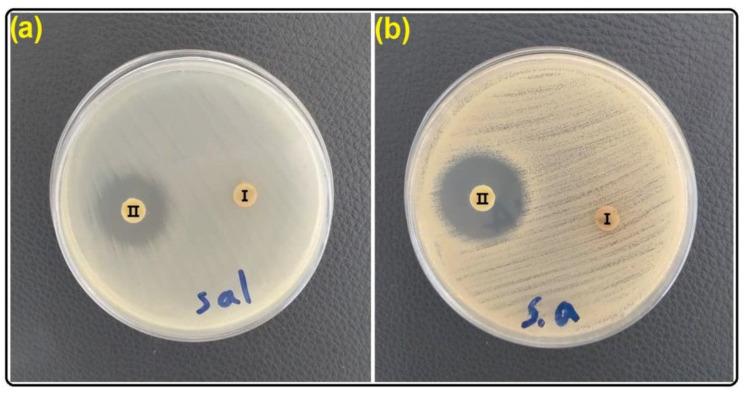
Antibacterial activity of (I) SGC and (II) SGC/3% CT-MMT: (a) *Salmonella paratyphi-A serotype* (b) *Staphylococcus aureus*.
